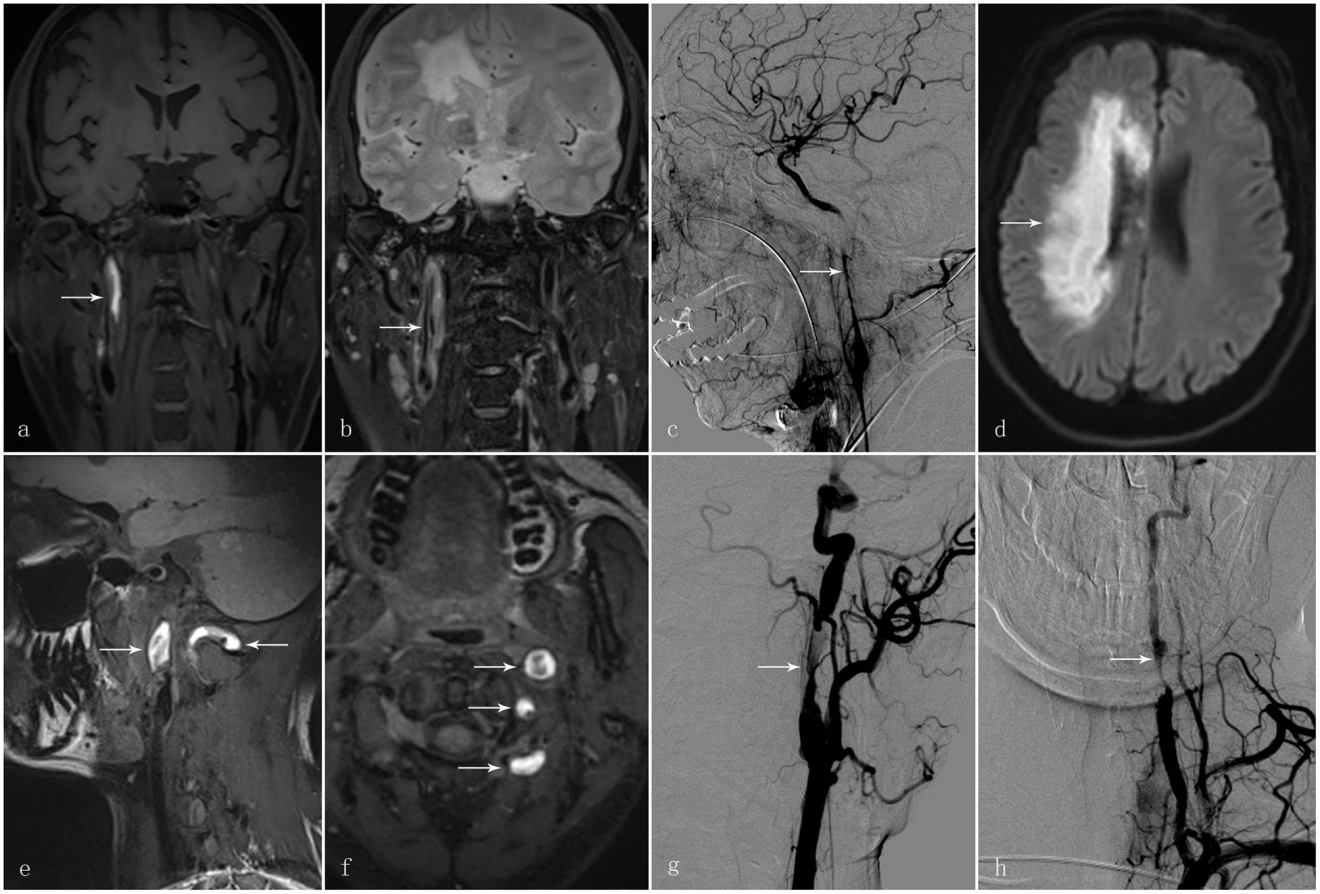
A 58-year-old man presented with sudden-onset left hemiparesis and paraesthesia. (a) Coronal view of the high-resolution MRI T1 SPACE images showed an intramural hematoma at the C1 segment of the right internal carotid artery [(a); arrow]. (b) T2 images demonstrated intimal flap and double lumen in the corresponding vessel wall [(b); arrow]. (c) Digital subtraction angiography in the same patient showed long tapering stenosis at the C1 segment of the right internal carotid artery [(c); arrow]; (d) Diffusion-weighted imaging demonstrated an acute infarct in the territory of the right internal carotid artery [(d); arrow]. Another 30-year-old man presented with vertigo and vomiting after cervical manipulation. (e, f) The high-resolution MRI T1 SPACE images showed the intramural hematoma at the left internal carotid artery and vertebral artery [(e, f); arrows]. (g) Digital subtraction angiography displayed irregular long tapering stenosis at the C1 segment of the left internal carotid artery and V2 segment of the left vertebral artery [(g, h); arrow].
